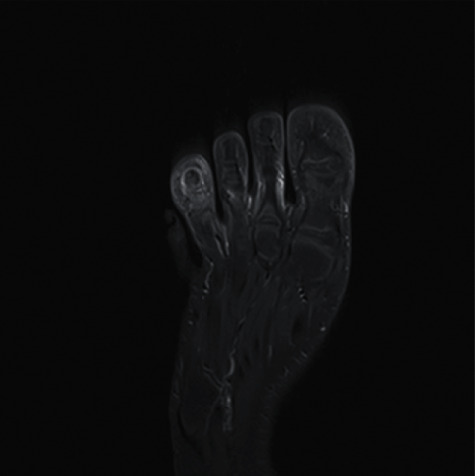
Gadolinium-enhanced T1-weighted images on MRI, showing the relative avascular nidus surrounded by hypervascular inflamed tissues.
